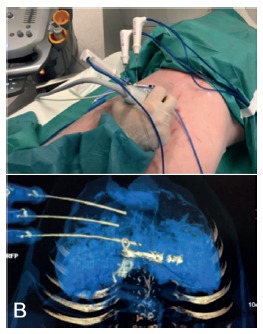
A) Placement the RFA needle using fusion image between US and CT; B) CT scan checking the correct position of the needles
